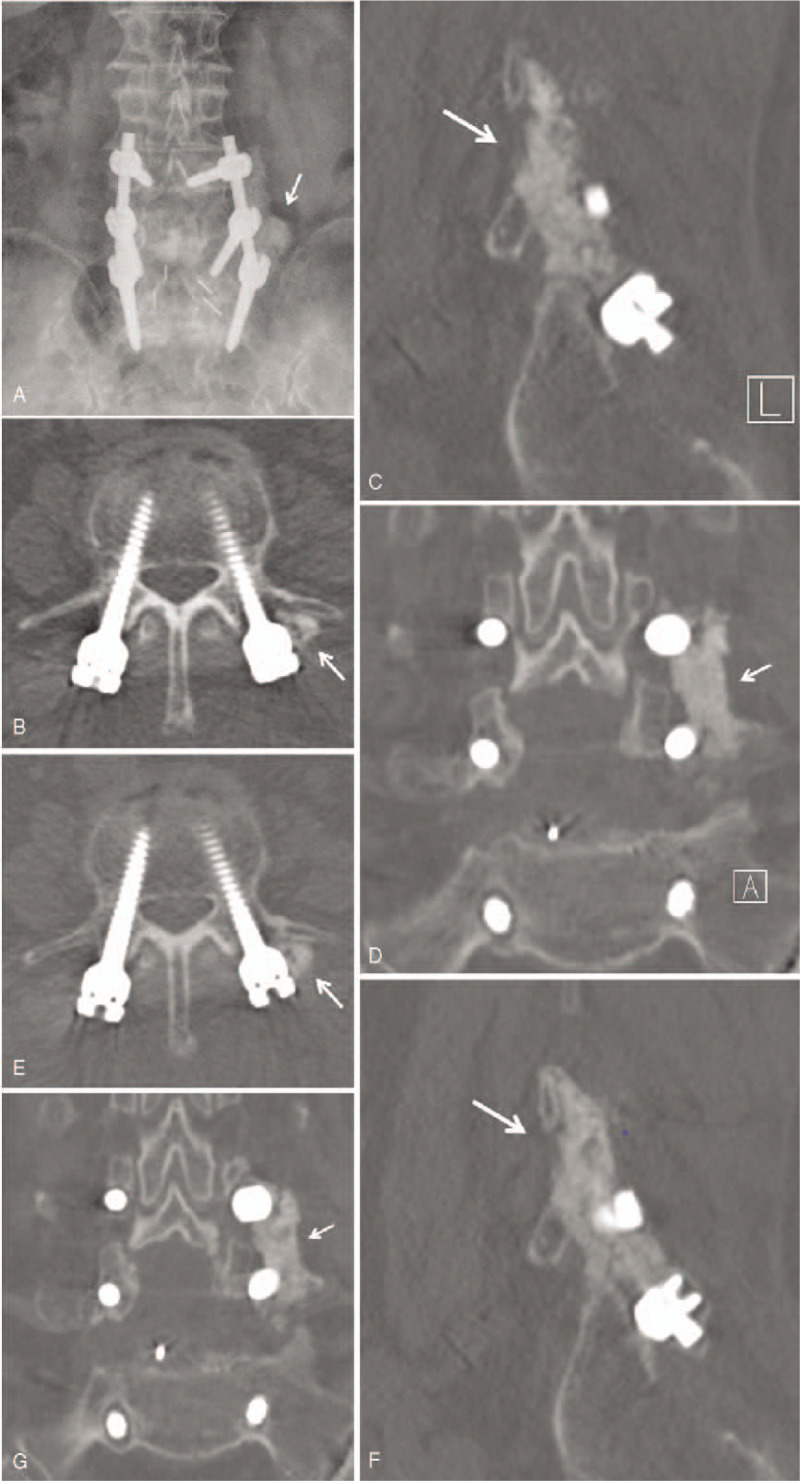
(A) A 76-year-old woman underwent decompression, transpedicular fixation, interbody fusion, and posterolateral fusion for degenerative spinal stenosis in L4-5, and isthmic spondylolisthesis (L5 on S1). Antero-posterior plain radiographs taken 6 months after surgery showed grade A fusion according to the Lenke classification on the left side (arrow). (B–D) Axial (B), sagittal (C), and coronal (D) CT images obtained 6 months after surgery showed grade A solid fusion. (E, F, G) Axial (E), sagittal (F), and coronal (G) CT images obtained 12 months after surgery showed grade A solid fusion.
